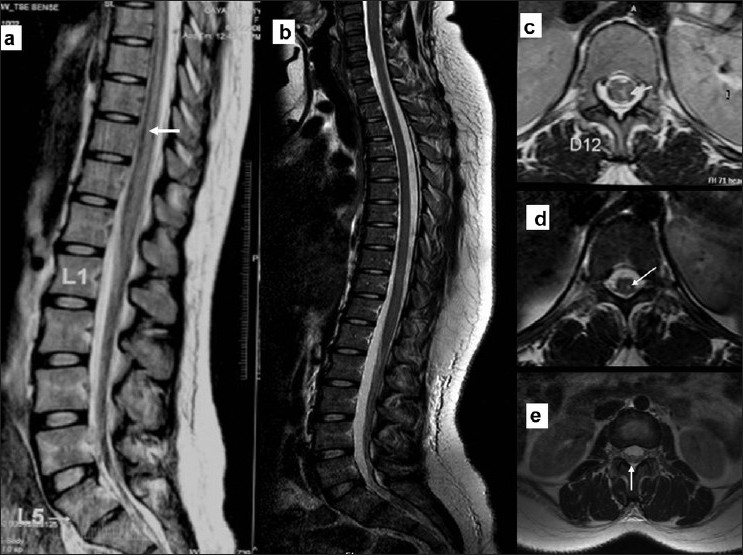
MRI immediately following neurologic deficits after spinal anesthesia and at 3 months follow-up. (a) T2-weighted (T2W) sagittal image of the lower dorsal and lumbosacral cord showing hyperintensities with cord edema at presentation; (b) sagittal T2W image at follow-up after 3 months showing decreased signal changes and edema; (c) T2W axial image of the lumbar cord demonstrating hyperintensity with cord edema at presentation; (d) T2W axial image of the follow-up scan after 3 months with hyperintensity within the cord with reduced cord edema; and (e) T2W axial image of the lumbar cord revealing clumped nerve roots suggestive of arachnoiditis 3 months after presentation.
